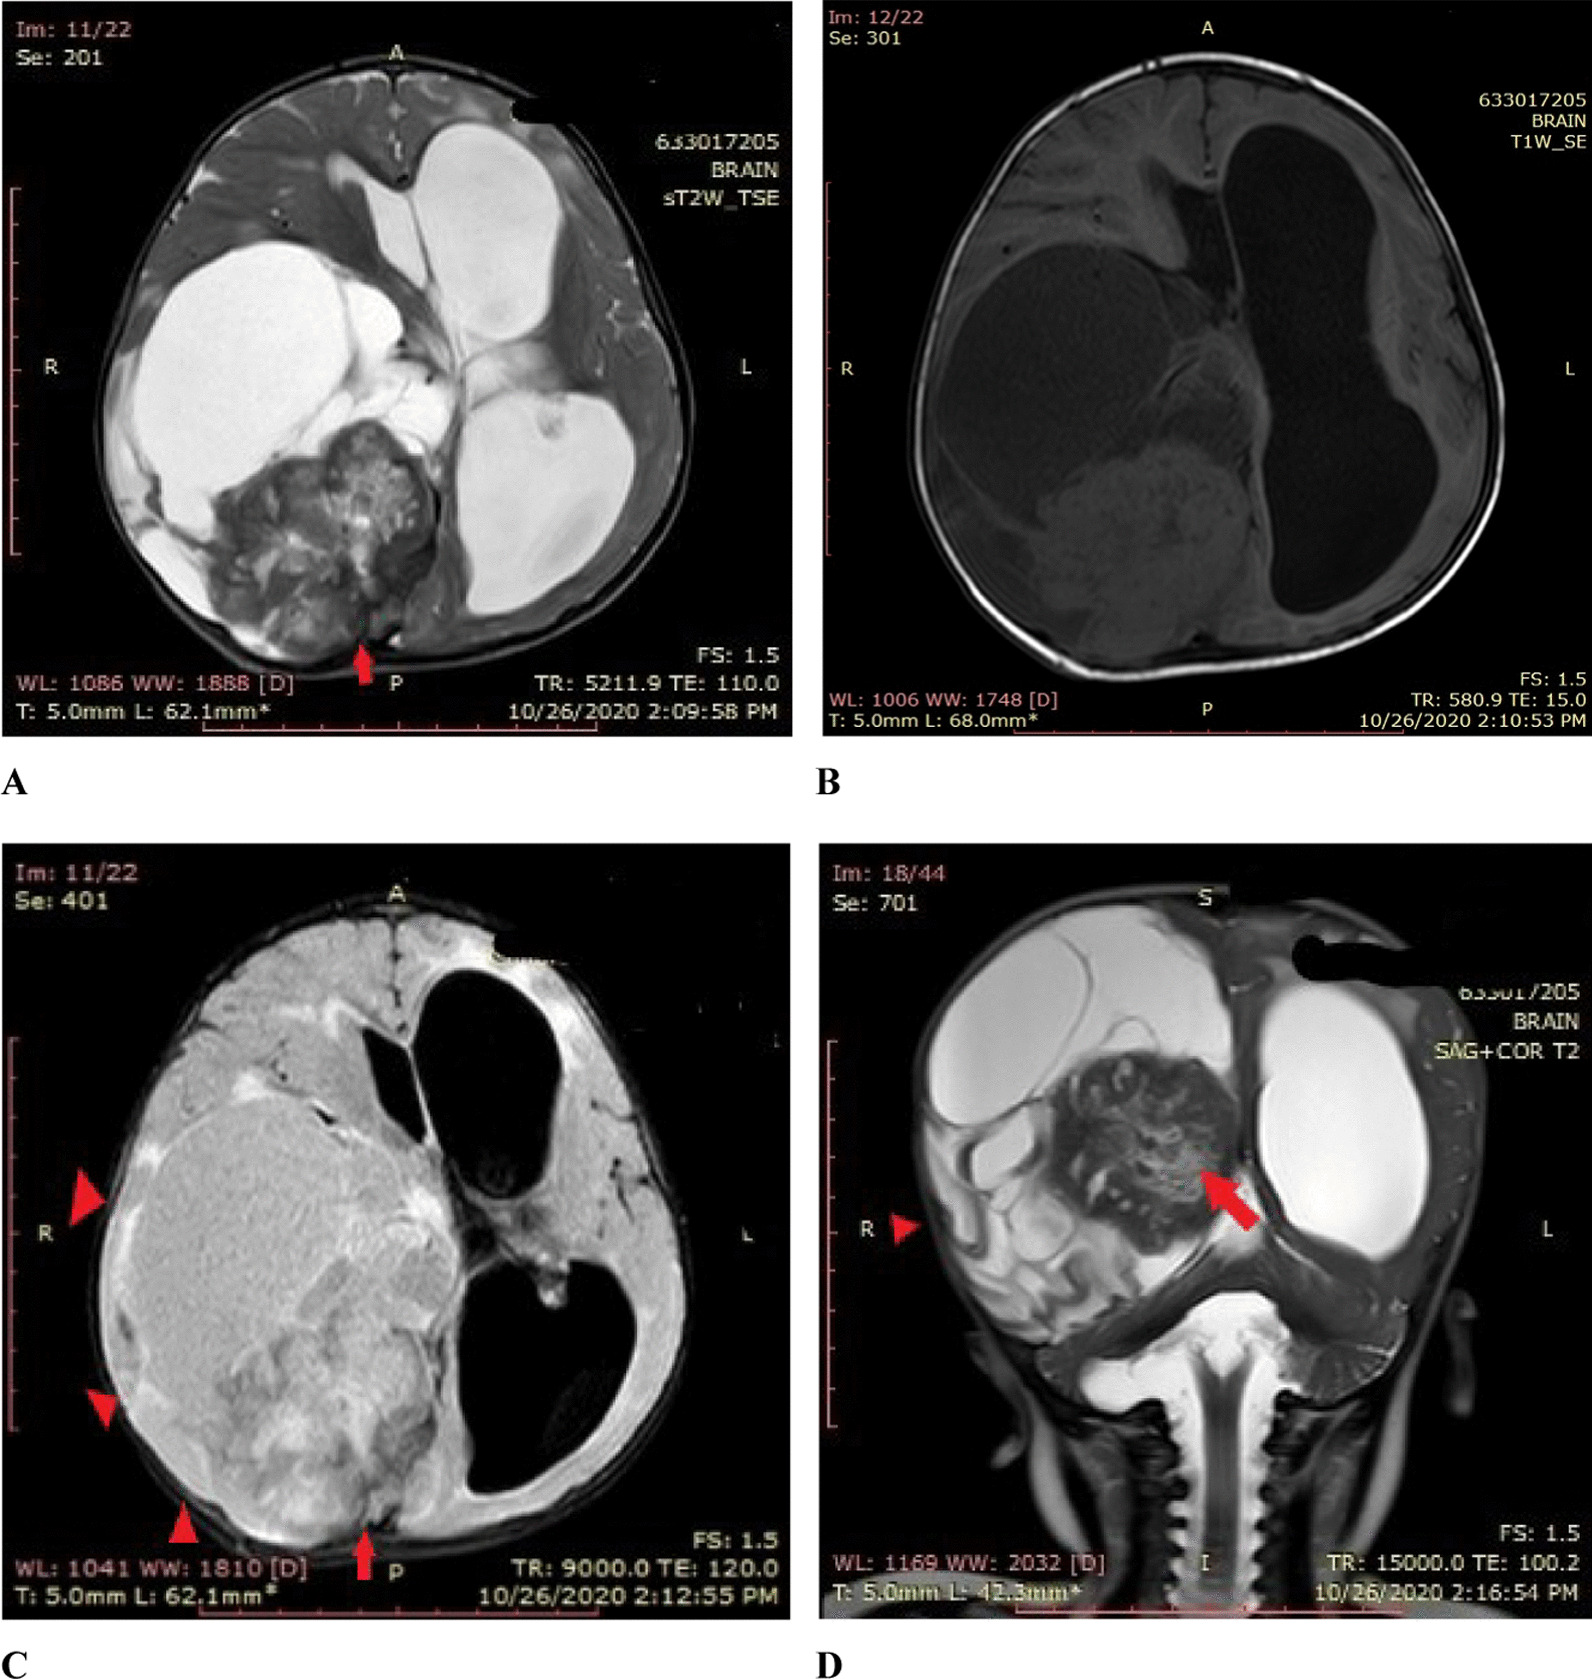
Pre-operative MRI without contrast. A–C Axial T2 W/T1W/FLAIR images show a heterogeneous intensity mass (red arrow shows solid component), (red arrowhead shows the cystic component) in the right parieto temporo occipital location compressing ipsilateral lateral ventricle causing a mild midline shift. D Coronal T2W images show a heterogeneous signal intensity mixed solid and cystic mass in right parieto temporo occipital location compressing the ipsilateral right lateral ventricle with obstructive hydrocephalus
